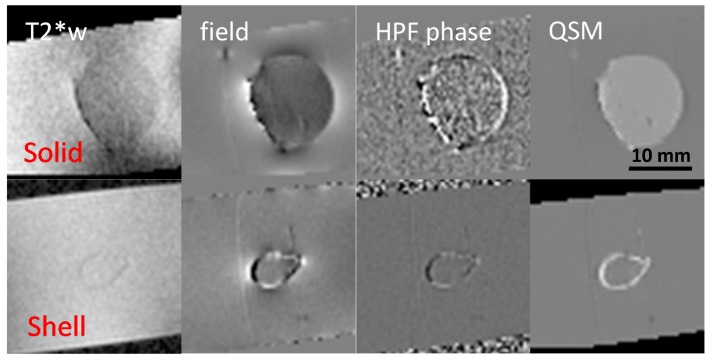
Solid and shell susceptibility (ultrasmall superparamagnetic iron oxide particles (USPIO)) object appearance in MRI techniques. Solid and shell lesions can be identified on QSM images; however, they both appear shell-like on phase images. (Source: [170]).
